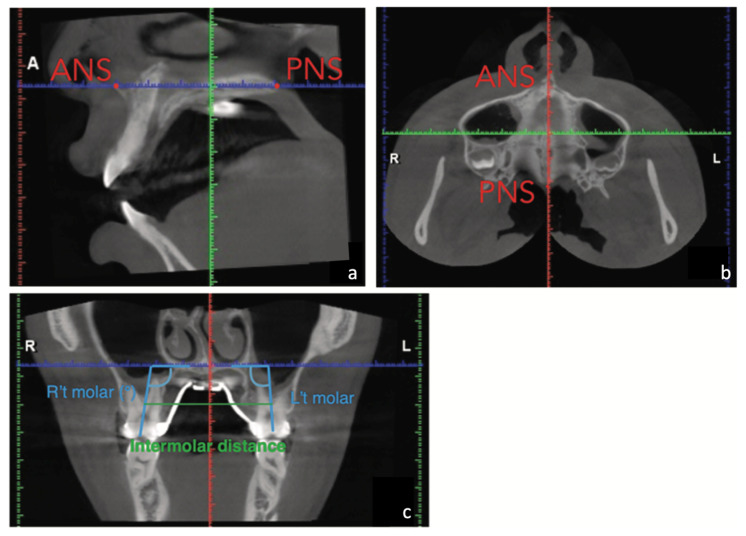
Head orientation of the cone beam computerized tomography (CBCT) images. (a) The sagittal plane was determined by the points of anterior nasal spine (ANS), posterior nasal spine (PNS), and the most anterior point of the vomer (V). (b) The axial plane was set perpendicular to the sagittal plane and parallel to the palatal plane (ANS-PNS). (c) The coronal plane was perpendicular to these two reference planes and passed through the level of the trifurcations of maxillary first molars.
